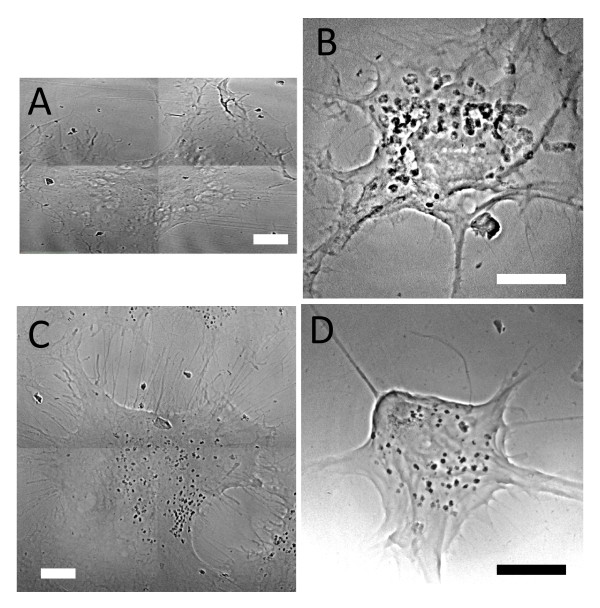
Transmission x-ray microimages images showing the different internalization of AuNPs by different cell lines. A) An EMT cell co-cultured with 1 mM PEG‐coated AuNPs for 48 h. B) An EMT cell co-cultured with 500 μM naked AuNPs for 48 h. C) A HeLa cell co-cultured with 500 μM naked AuNPs for 48 h. D) A CT-26 cell co-cultured with 500 μM naked AuNPs for 48 h. (Bars: 5 μm).
